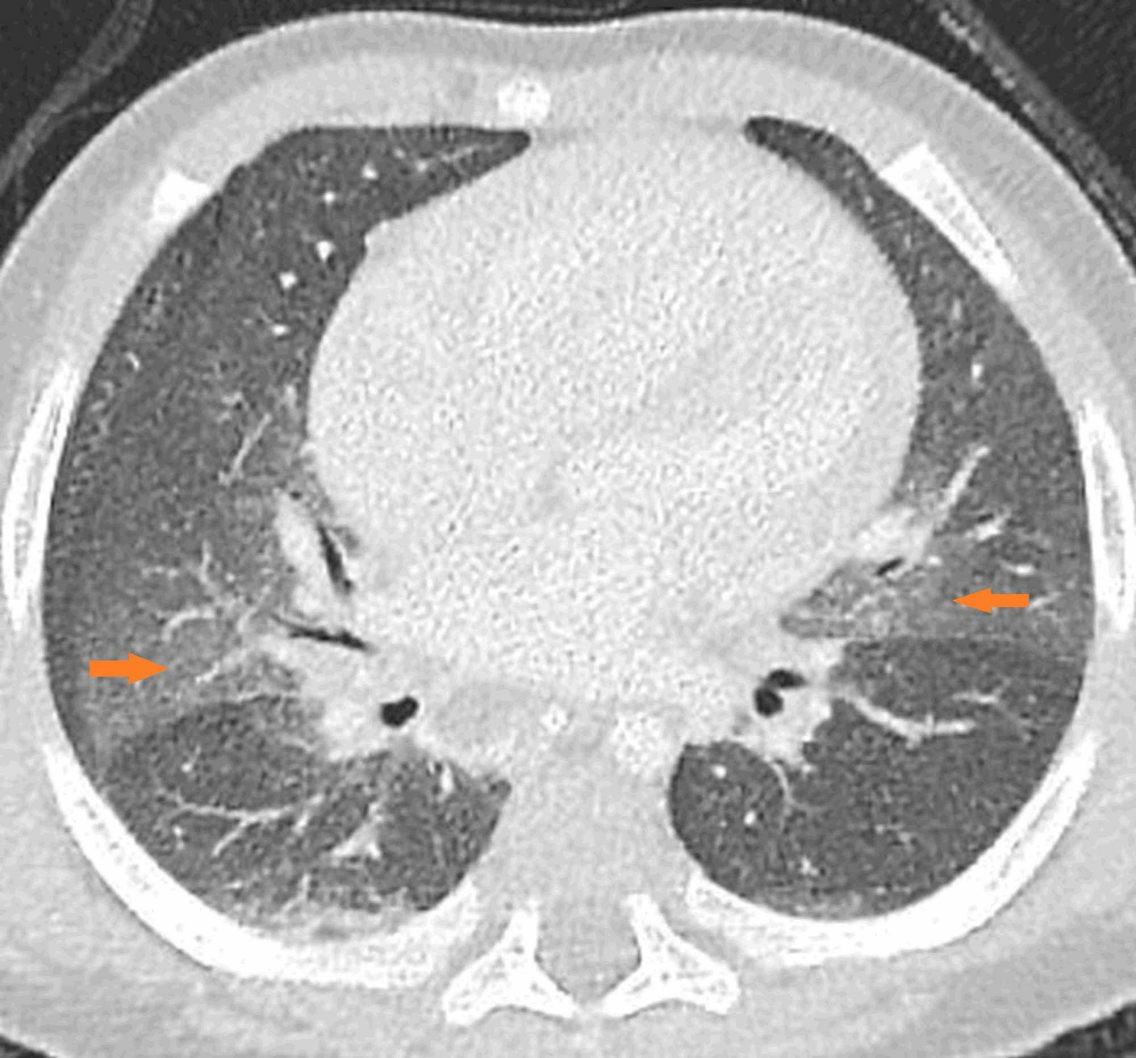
Axial HRCT section of the lung at the level of the middle lobe Arrows on both sides show the geographic ground-glass opacities. HRCT, high-resolution computed tomography
